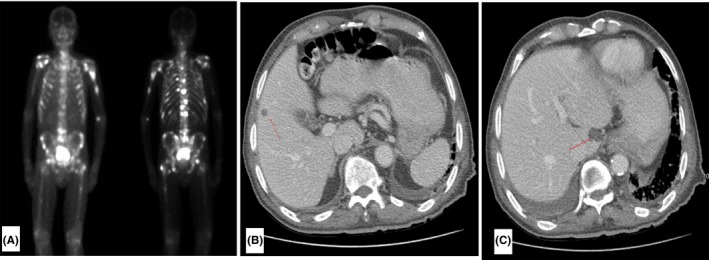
Skeletal metastases on bone scan (A) and no hepatobiliary metastases on contrast CT (B, C, arrows show benign cystic lesions in the liver) at the time of initial PCa diagnosis
